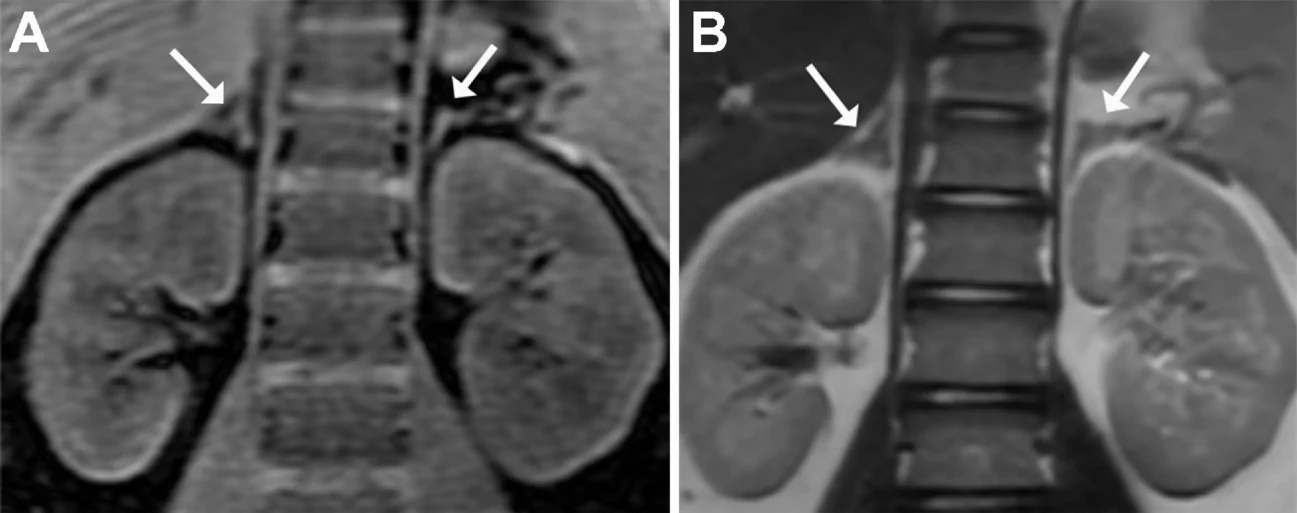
Normal adrenal glands on MRI of an 11-year-old female with secondary amenorrhea. Coronal (a) T1-weighted fat-suppressed and (b) T2-weighted single-shot fast-spin echo images show normal orientation and morphology of each adrenal gland (*arrows*), with an inverted Y-shape on the right and inverted V-shape on the left. Adrenal tissue is thin, without thickening, elongation, or mass. Signal is isointense to renal cortex on T1-weighted imaging and slightly hypointense to renal cortex on T2-weighted imaging
